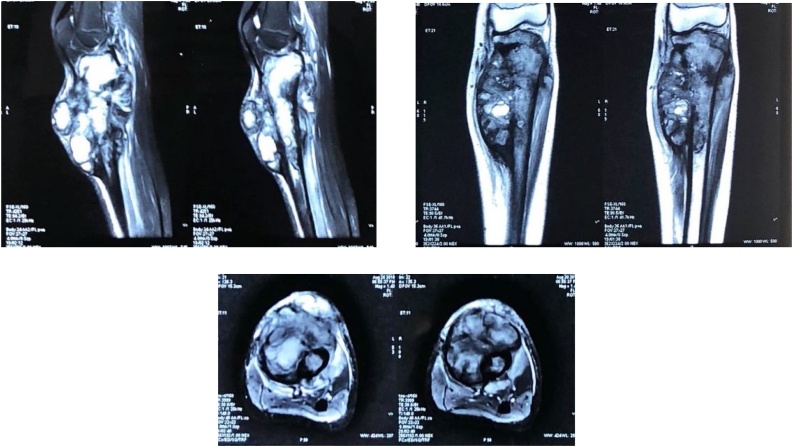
MRI showed a heterogenous mass in epiphysis extending to diaphysis of tibia, 16 cm from tibiofemoral joint, without neurovascular bundle involvement.
